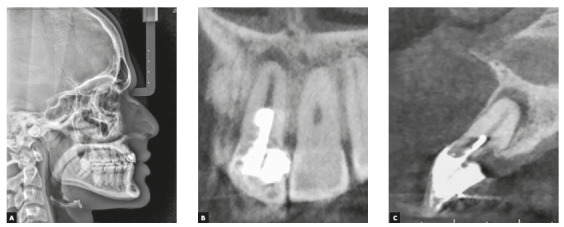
Final lateral radiograph and Cone Beam Computerized Tomograph image
